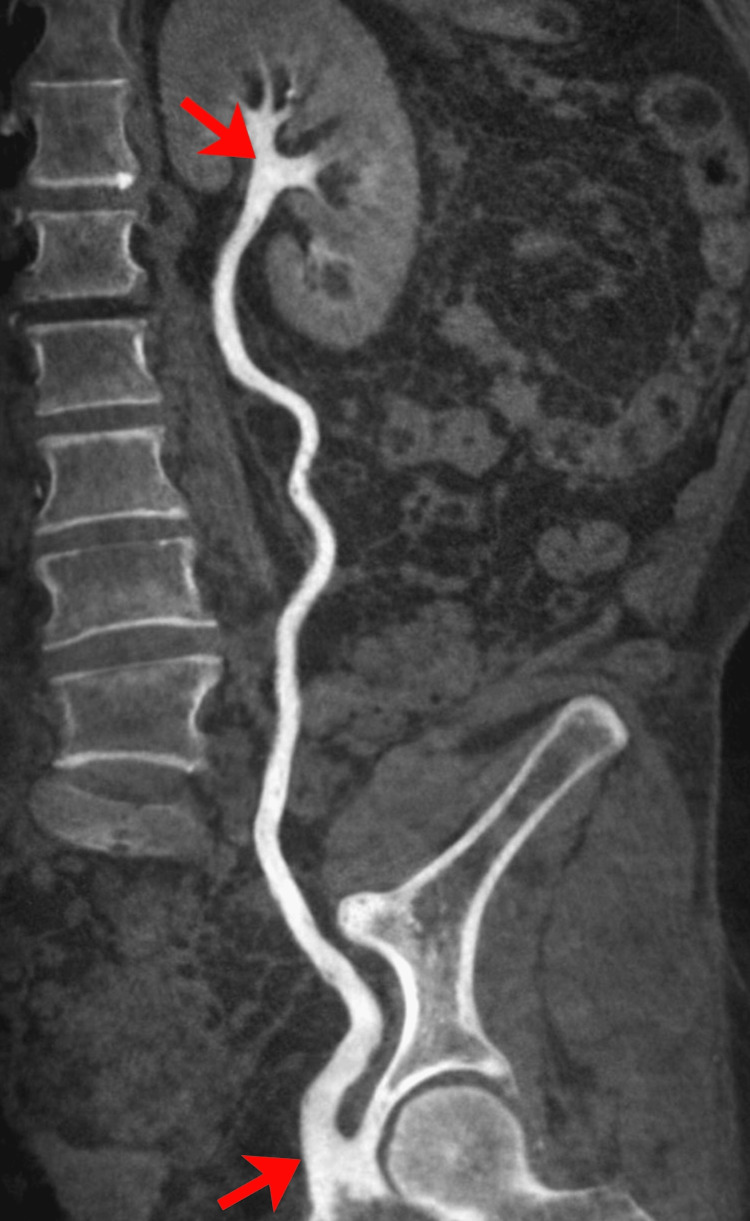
Contrast-Enhanced CT Urography Contrast-enhanced CT urography showing marked dilation of the right pelvicalyceal system (as indicated by the arrows). No calculus or surgical clip entrapment was identified.
